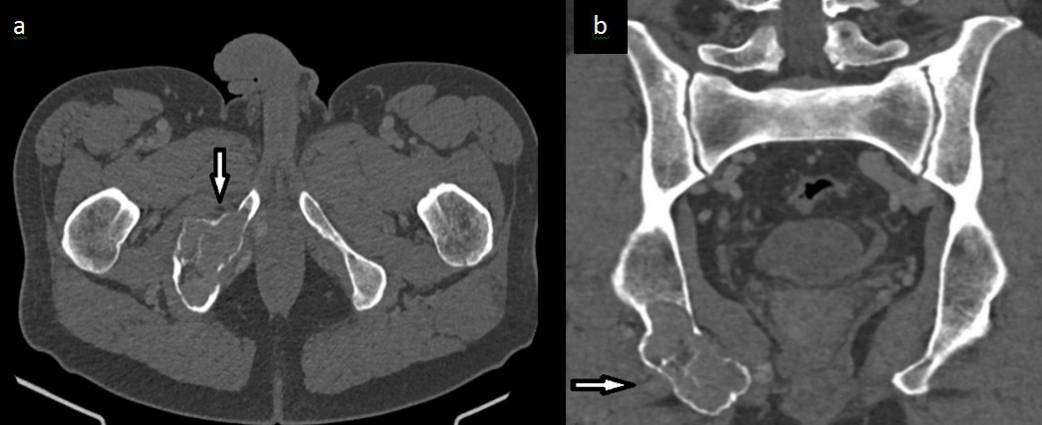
Axial and coronal pelvic CT scans in the bone window showing an osteolytic lesion of the right ischio pubic ramus (arrow) destroying the underlying cortical bone, without periosteal reaction.
